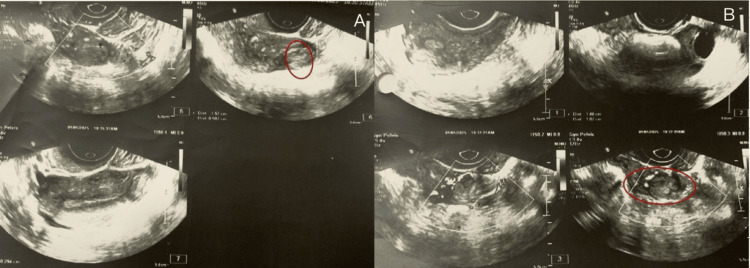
(A) Transvaginal ultrasound: Lesion regressed on day 7 after third dose of methotrexate. (B) No blood flow within the lesion on color Doppler with satisfactory response to methotrexate
